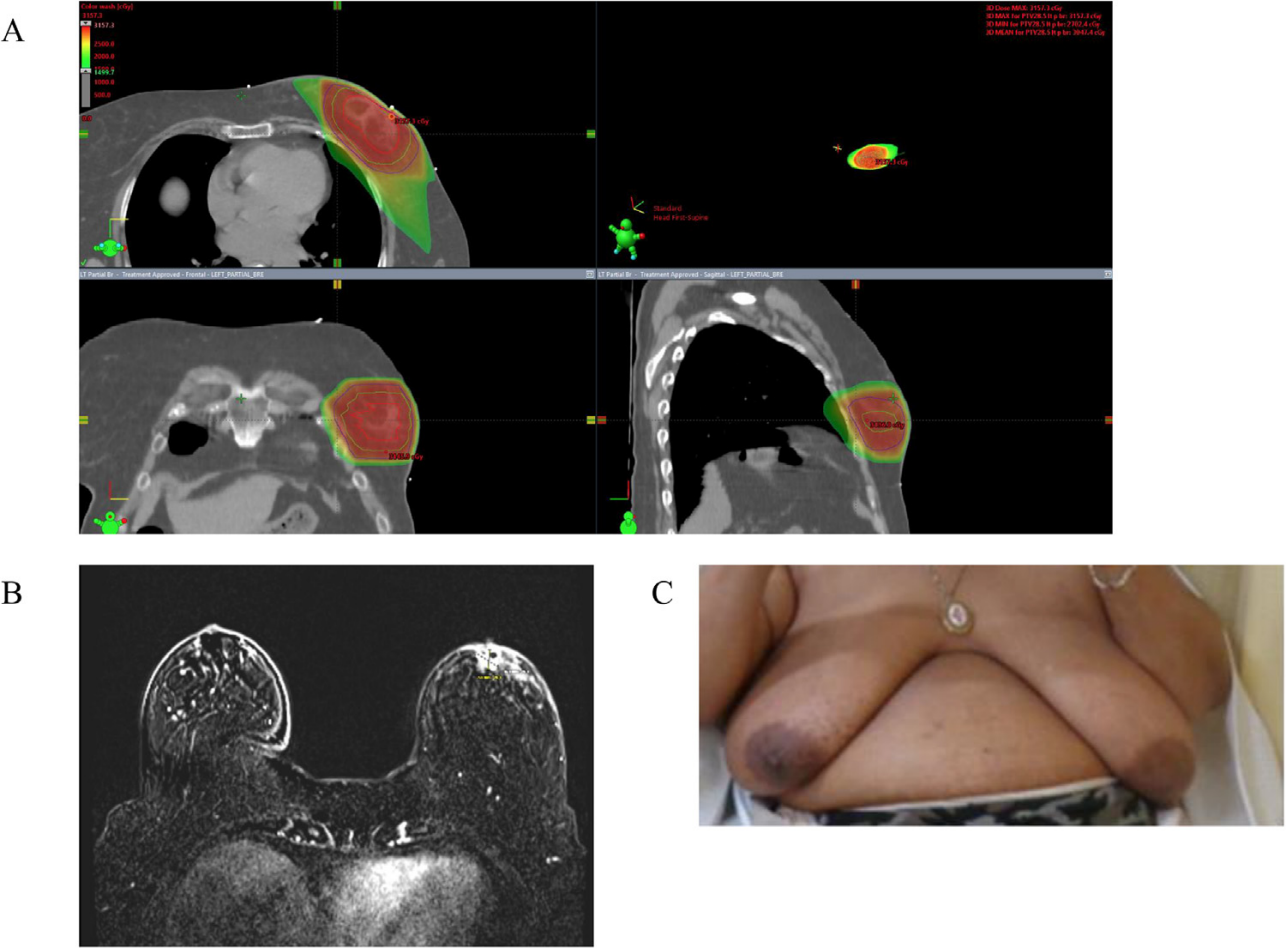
Radiation, MRI, and photographic imaging eliciting oncoplastic reconstruction. (A) Radiation dose distribution in a patient treated with lumpectomy with oncoplastic reconstruction. The tumor bed is contoured in red, the PTV30 in cyan, and the PTV28.5 in dark blue. (B) Bilateral breast MRI with contrast demonstrates subareolar enhancing mass to assist with target delineation despite oncoplastic reconstruction. (C) Photographic example of an excellent physician- and patient-reported cosmetic outcome despite a large right breast PTV30 volume of 397 cc. *Abbreviations:* MRI = magnetic resonance imaging; PTV = planned tumor volume.
